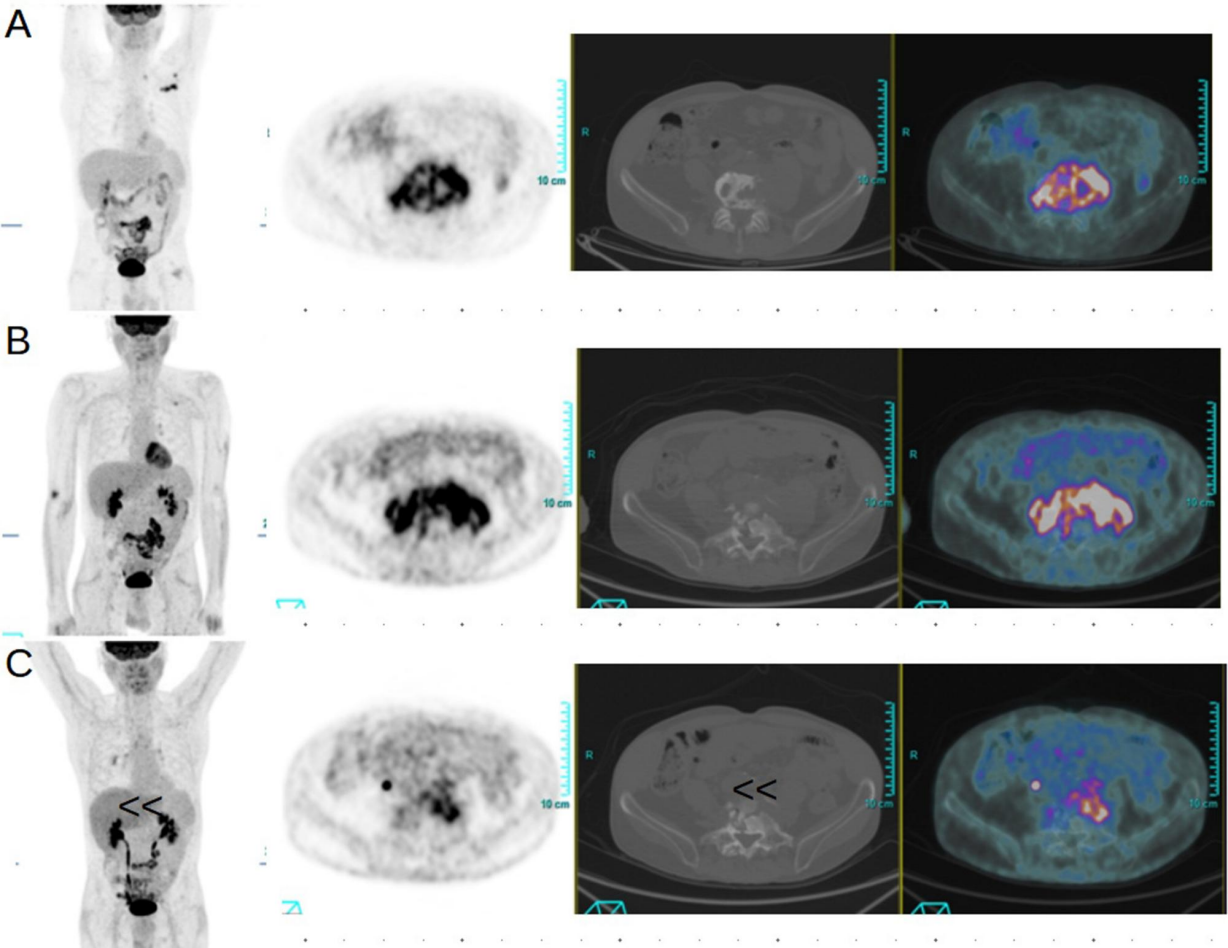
A 60-year-old man with back pain and increased inflammatory markers executed 2-[^18^F]FDG PET/CT showing the presence of an increased uptake in the column corresponding to the fourth and fifth lumbar vertebra. Blood cultures were positive for Staphylococcus aureus. Maximum intensity projection PET (A), axial PET, and fused PET/CT images confirmed the increased 2-[^18^F]FDG uptake in the intervertebral disk and adjacent vertebra suspected for spondylodiscitis. After 3 months of antibiotic treatment, a new 2-[^18^F]FDG PET/CT (B) confirmed the previous uptake increased in extension and intensity demonstrating the lack of effectiveness of therapy. Thus, the patient started a new antibiotic regimen with partial metabolic response demonstrated in the subsequent 2-[^18^F]FDG PET/CT (C) performed after 2 months.
